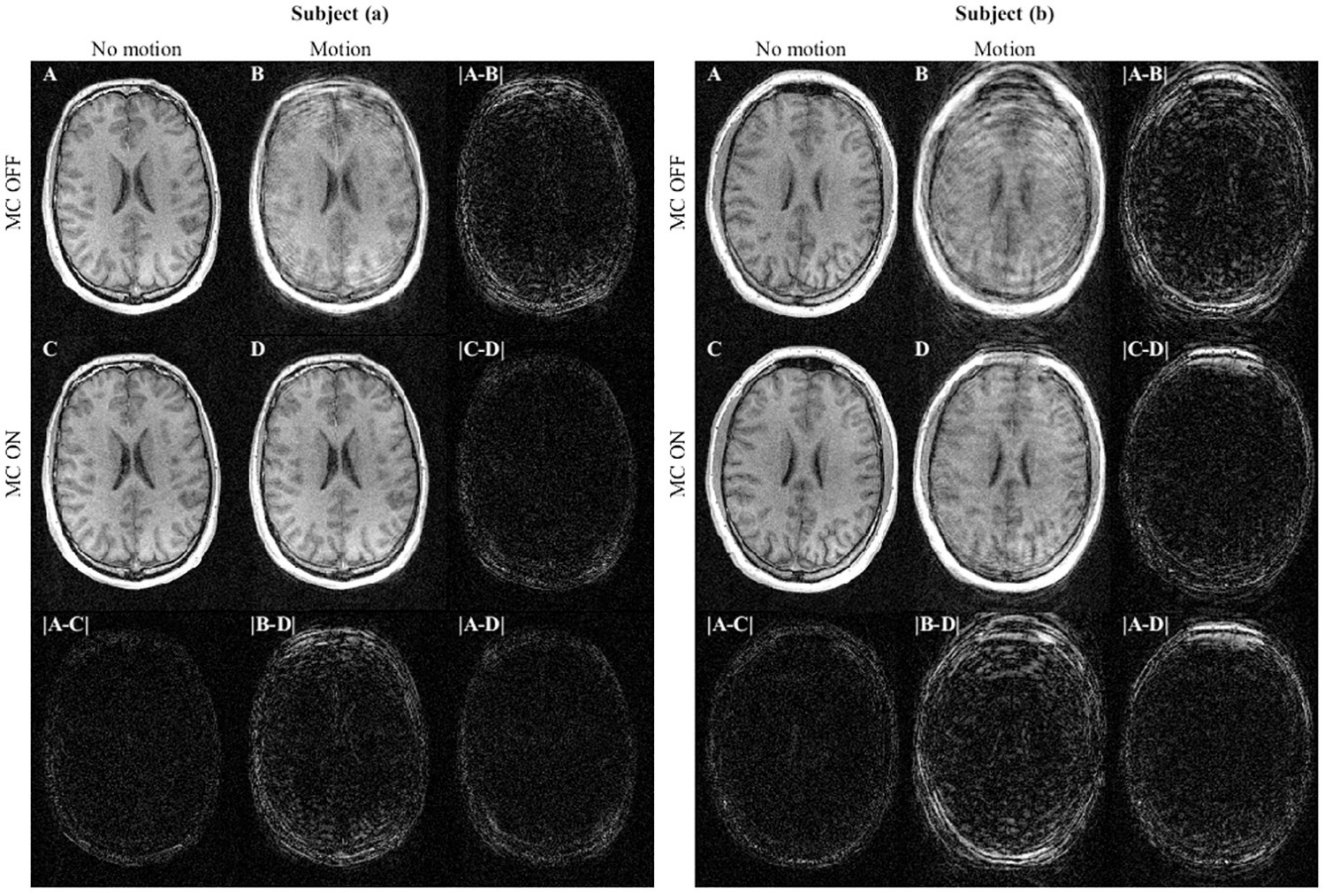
Axial image slices from the 3D FLASH scans of subjects (a) and (b). The motion performed during the acquisition corresponds to the graphs in Fig 6 subject (a) and (b), respectively. The four scan images representing scans with and without motion and with and without motion correction (MC): A: *No motion, MC OFF*, B: *Motion, MC OFF*, C: *No motion, MC ON*, and D: *Motion, MC ON*. The absolute difference between scans are present in the last row and column for both subjects. These are visualized using the same mapping from pixel intensities to grey scale values as in the MRI images. For both subjects image B show degraded image quality resulting from motion. Image D shows the improved image quality as a result of the prospective MC.
